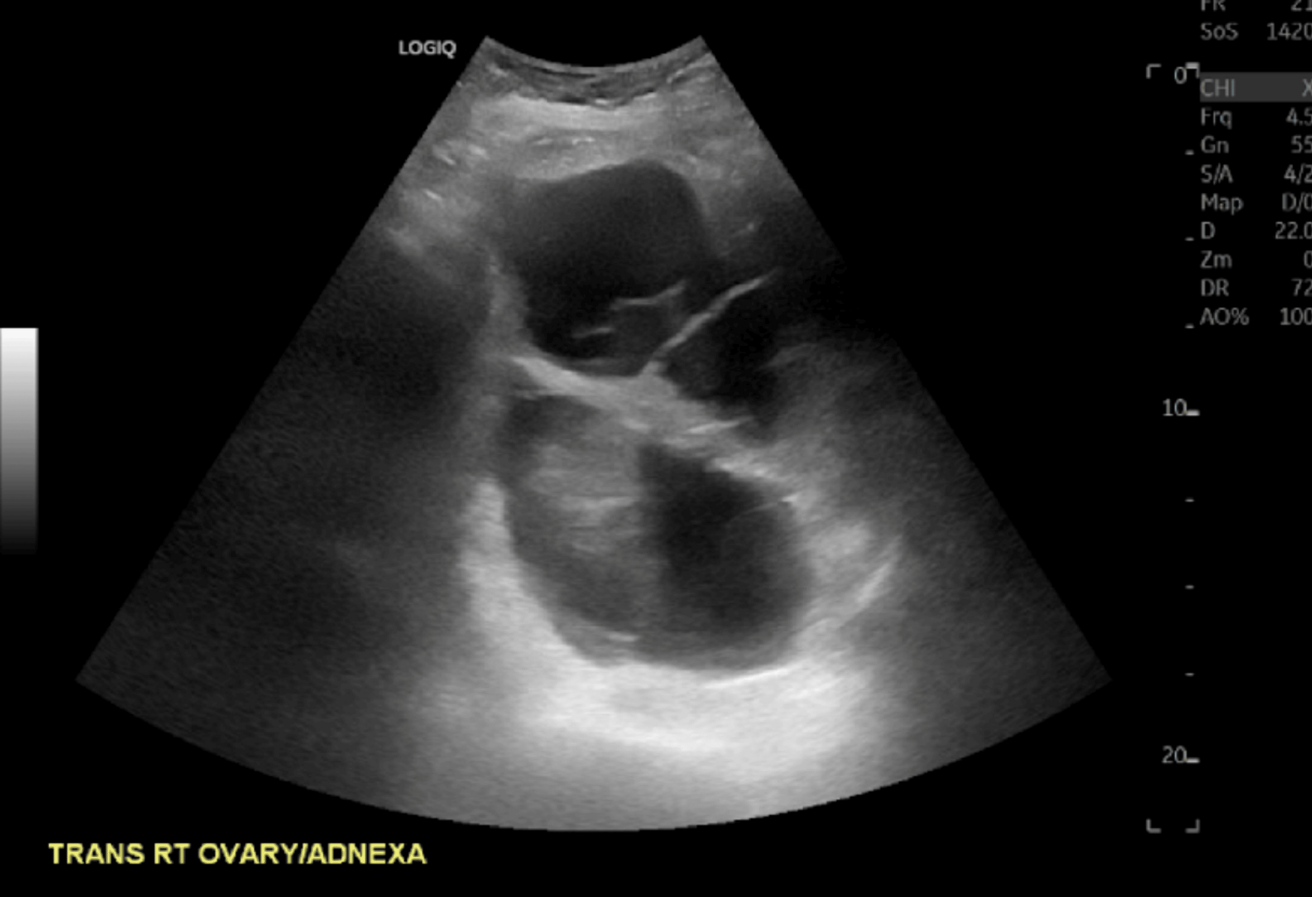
Pelvic ultrasound showing a large multicystic right ovary
